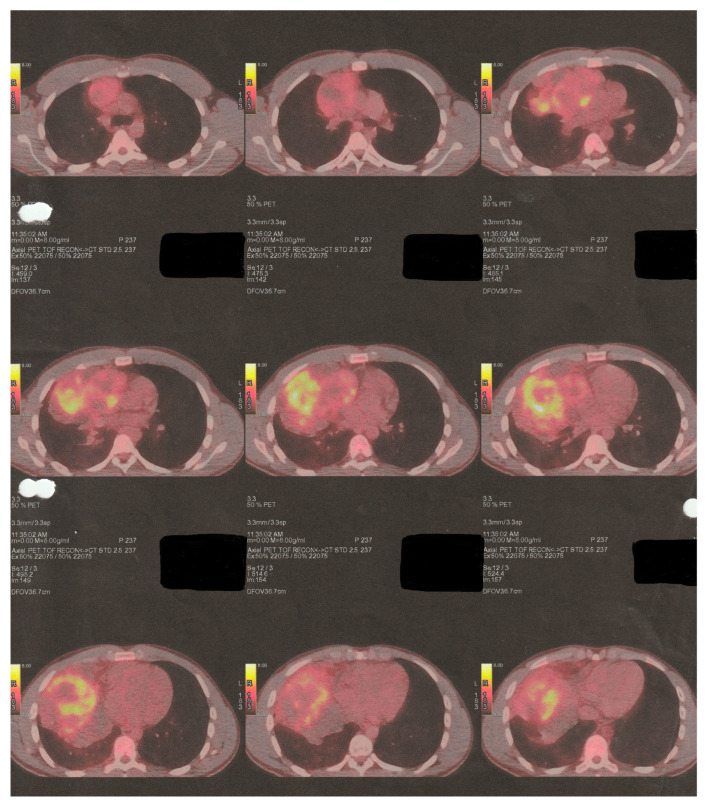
Initial preoperative PET-CT imaging control.
